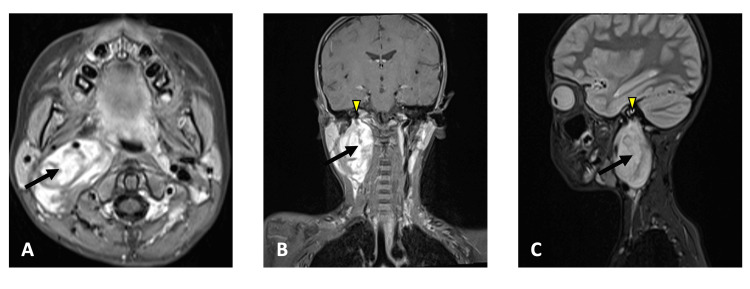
Axial (A), coronal (B), and sagittal (C) contrast-enhanced MRI demonstrating a tumor (black arrow) within the parapharyngeal space extending superiorly toward the skull base (arrowhead).
